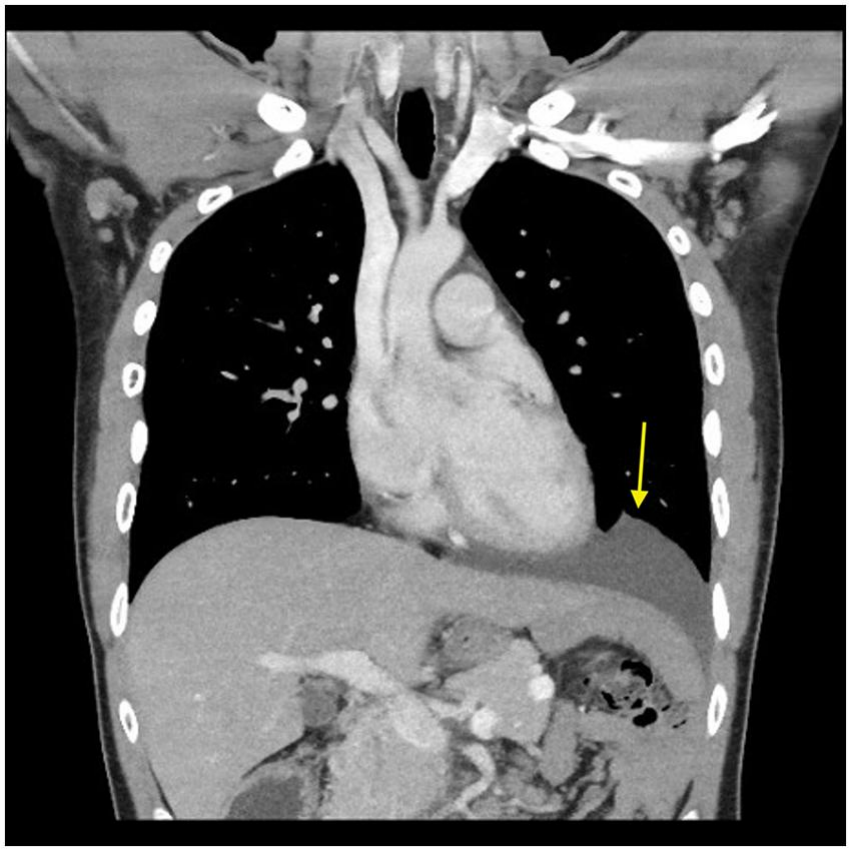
Postoperative CT scan of the chest, abdomen, and pelvis with contrast, coronal view. Showing reduced previously herniated abdominal organ into the left hemidiaphragm (arrow).
